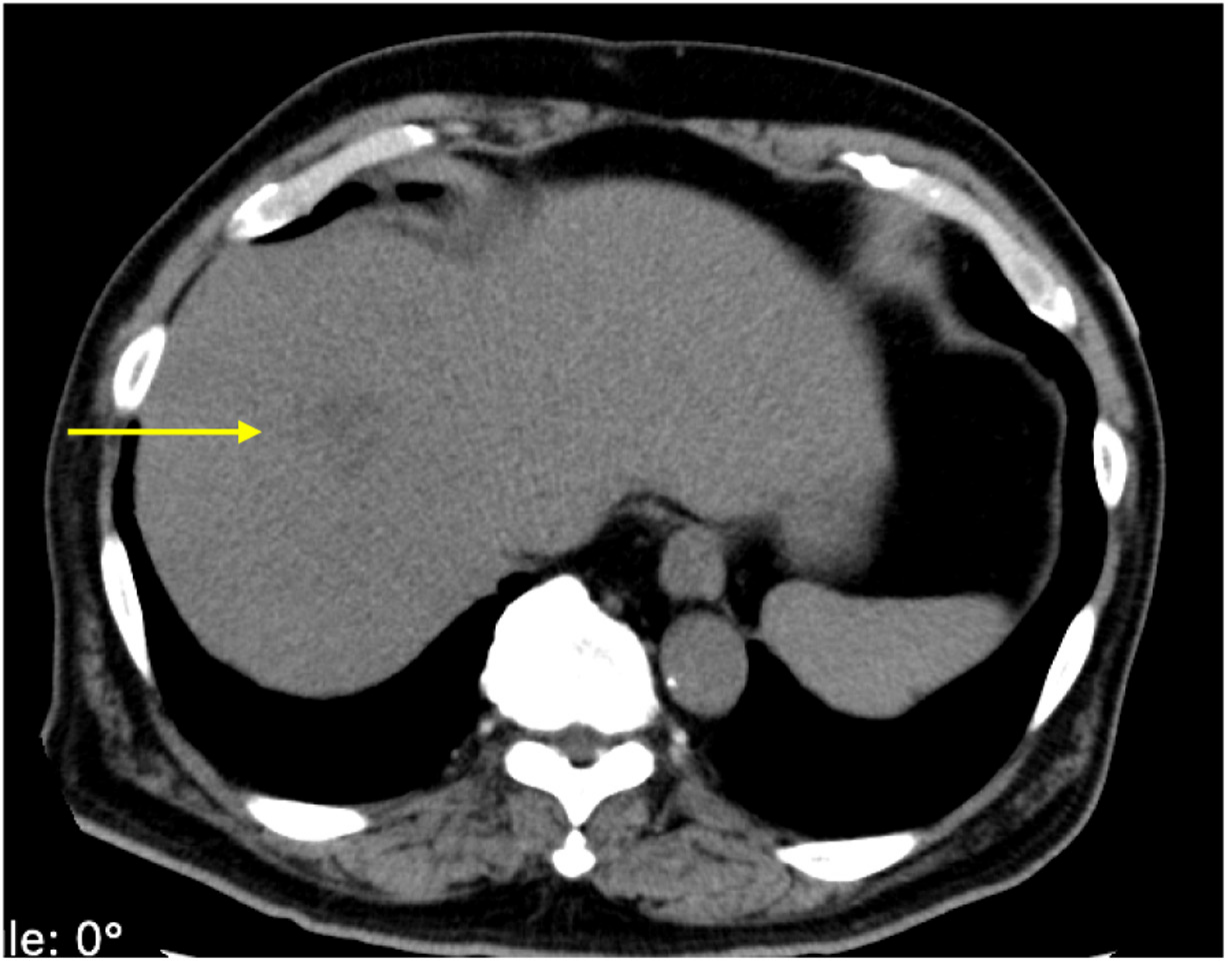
Abdominal CT scan without contrast‐enhancement on admission reveals a 26‐mm low‐density lesion.
